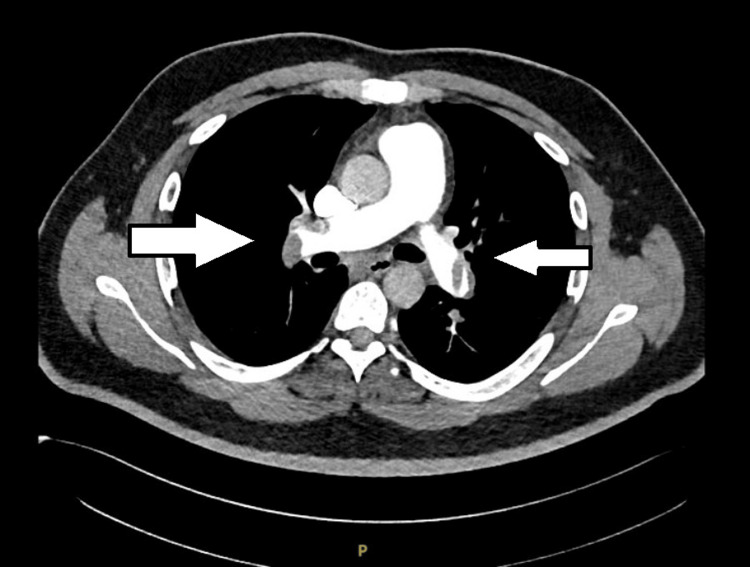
The patient's CT pulmonary angiography (axial view) shows multiple filling defects in superior and inferior segmental branches of both pulmonary arteries, suggestive of acute pulmonary thromboembolism.
